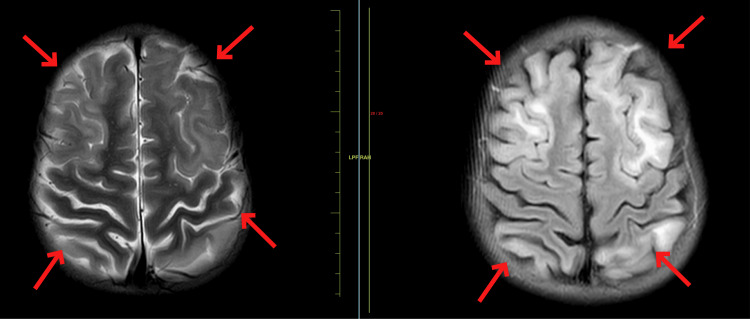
MRI showing T2/FLAIR hyperintensities in cortical and subcortical areas of bilateral fronto-parietal lobes. MRI: Magnetic resonance imaging; FLAIR: fluid-attenuated inversion recovery
